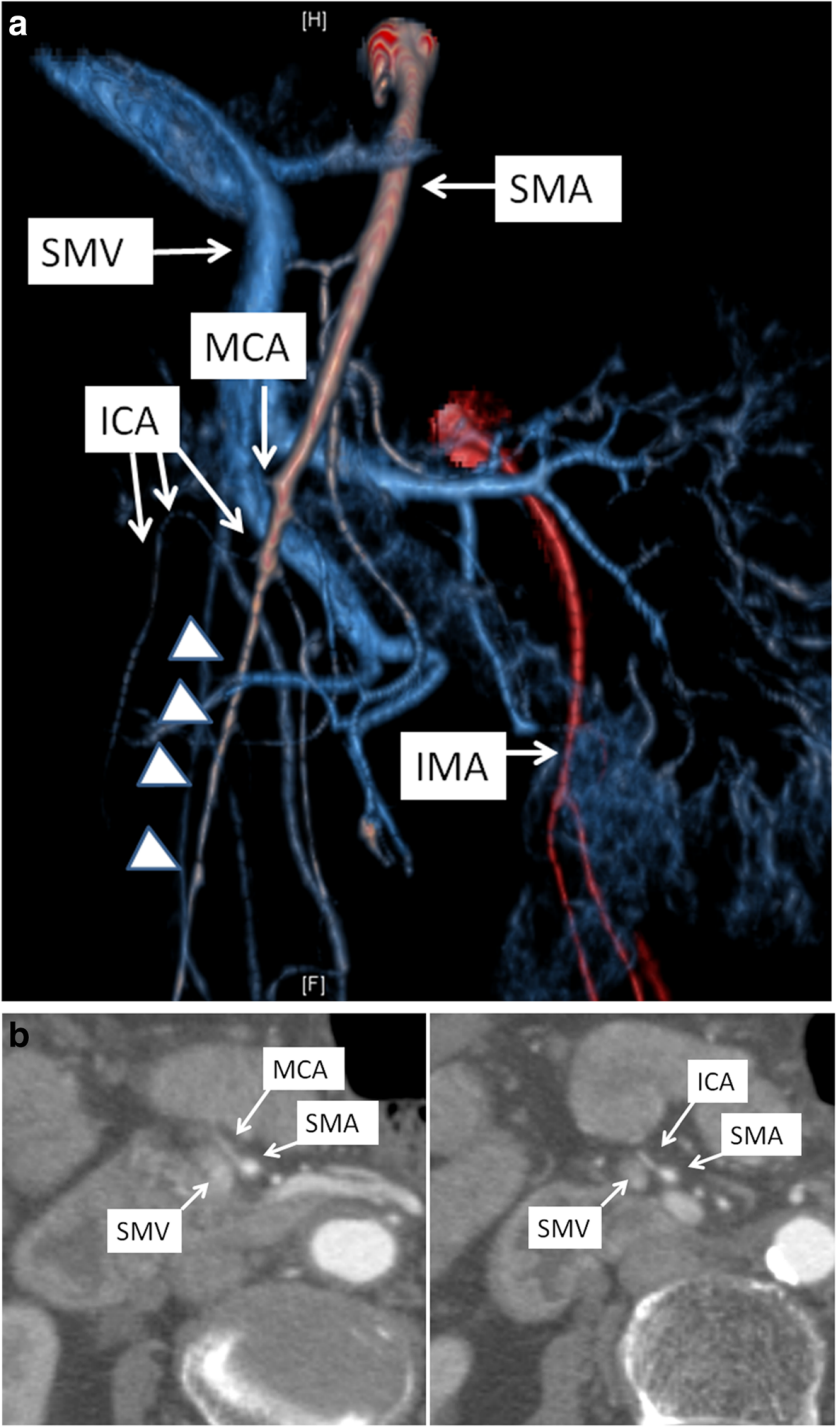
a Front view of the CT angiography. The irregularities of the SMA due to vasospasm were observed. The MCA could not be detected by CT angiography, and the narrowing of the ICA was observed. The RCA could not be detected. Although the IMA was patent, the left colic artery was also undetectable. There was no abnormality in the SMV. b In 2D axial view, compared to the SMV, narrowing of the SMA, MCA, and ICA was observed. No thrombosis was detected in the SMA and SMV. *ICA* ileocolic artery, *MCA* middle colic artery, *IMA* inferior mesenteric artery, *SMA* superior mesenteric artery, *SMV* superior mesenteric vein
